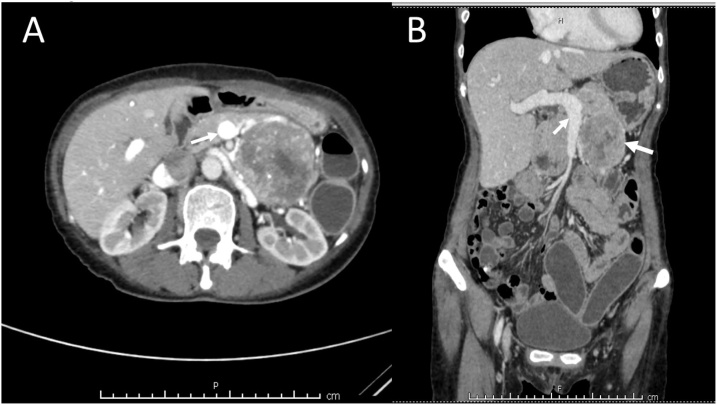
Axial (A) and coronal (B) view of contrasted abdominal CT showed heterogeneously enhanced solid-cystic tumor (larger arrow) abutted onto a great vessel (smaller arrow).
